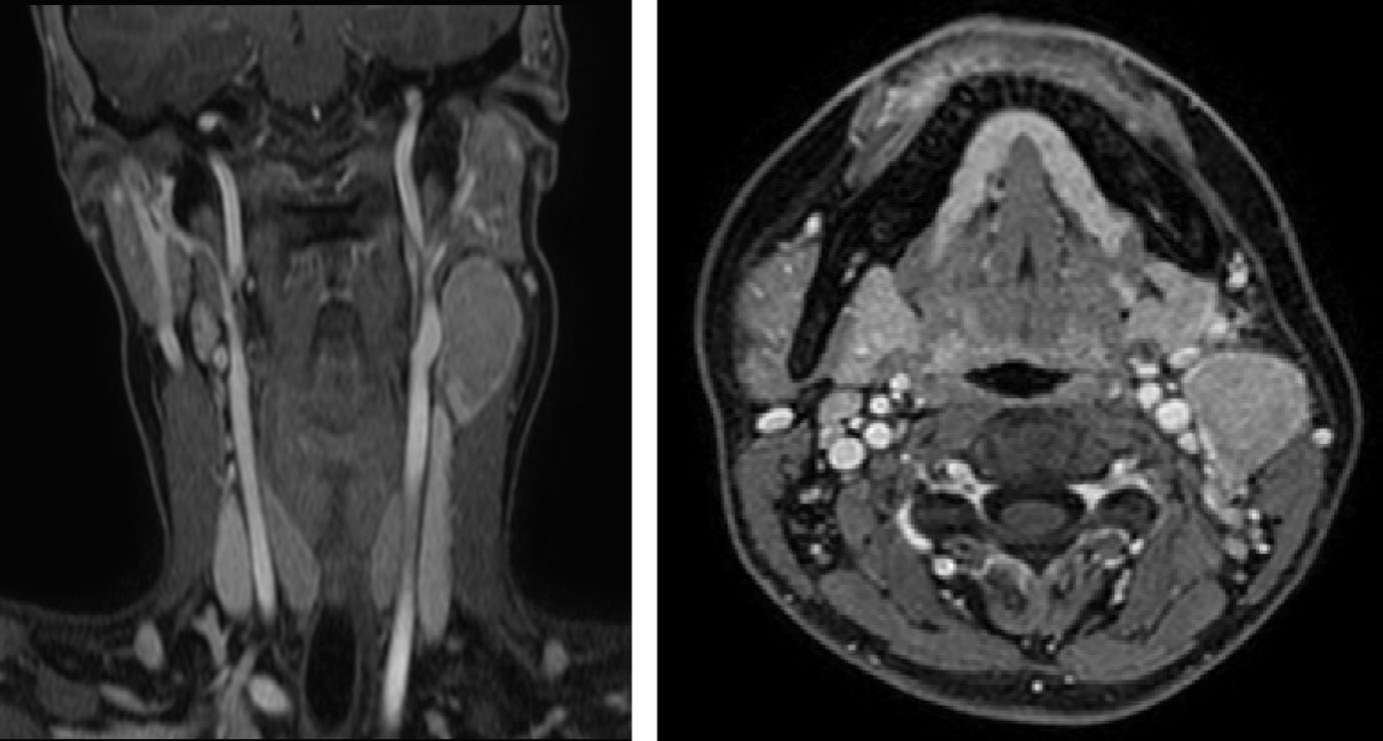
MRI coronal and axial scans show an ovoid mass with peripheral contrast enhancement causing displacement of surrounding musculature and vascular structures
